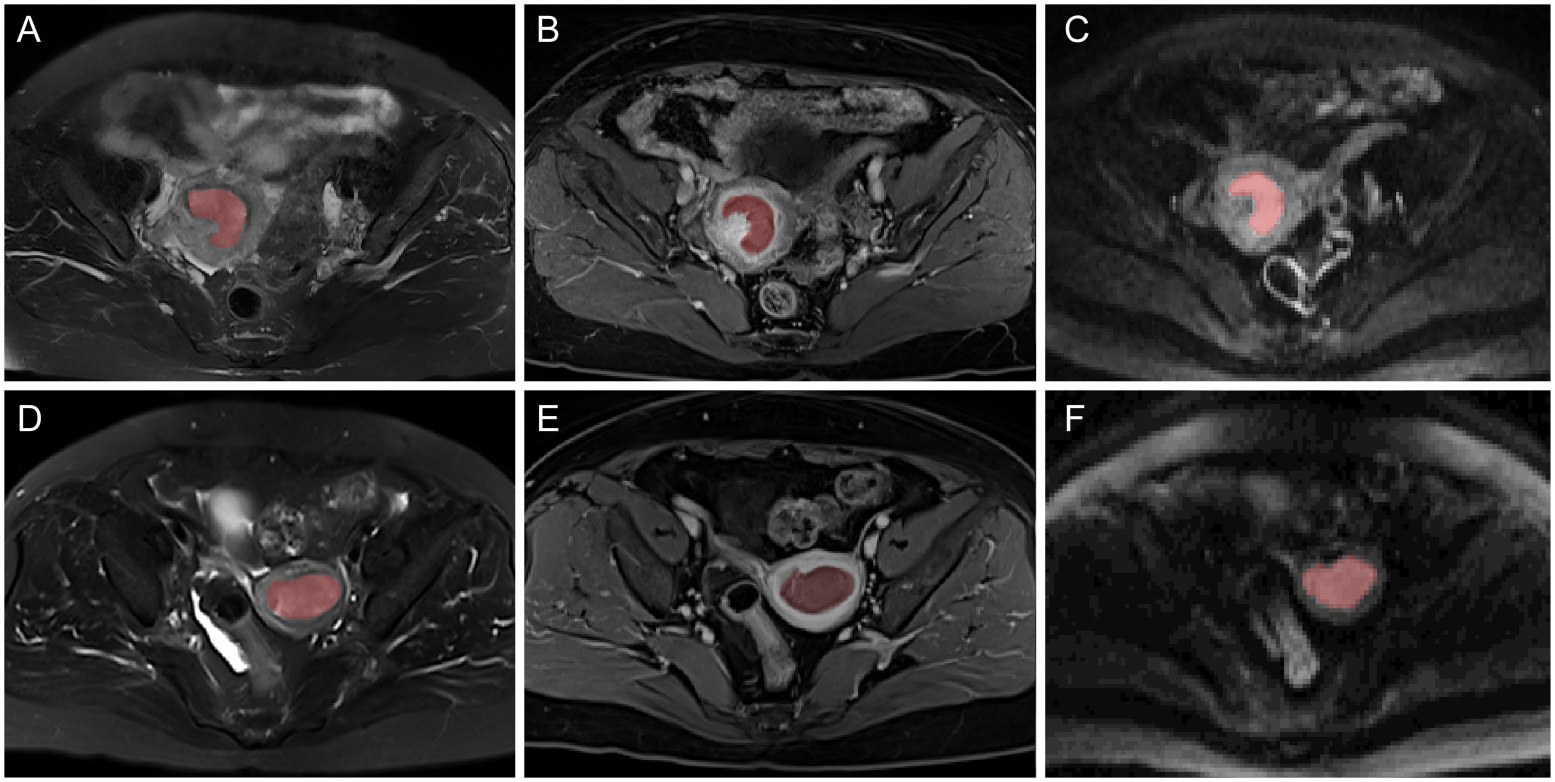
Typical multiparametric MR images of two EC patients with MSI-H (A–C) and MSI-L/MSS (D–F). From left to right: axial T_2_WI, CE-T_1_WI, and DWI MR images and the corresponding region of interest (ROI).
